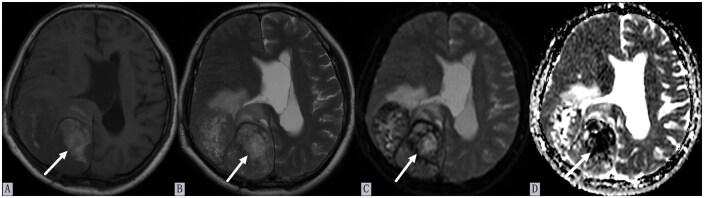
Brain MRI findings at 2-month follow-up. (A) Axial T1WI showing a well-defined right temporo-parieto-occipital mass with intrinsic T1 hyperintensity (arrow). (B) Axial T2WI demonstrating T2 hyperintensity within the mass (arrow). (C) DWI revealing restricted diffusion in the lesion (arrow). (D) ADC map confirming corresponding hypointensity (arrow).
